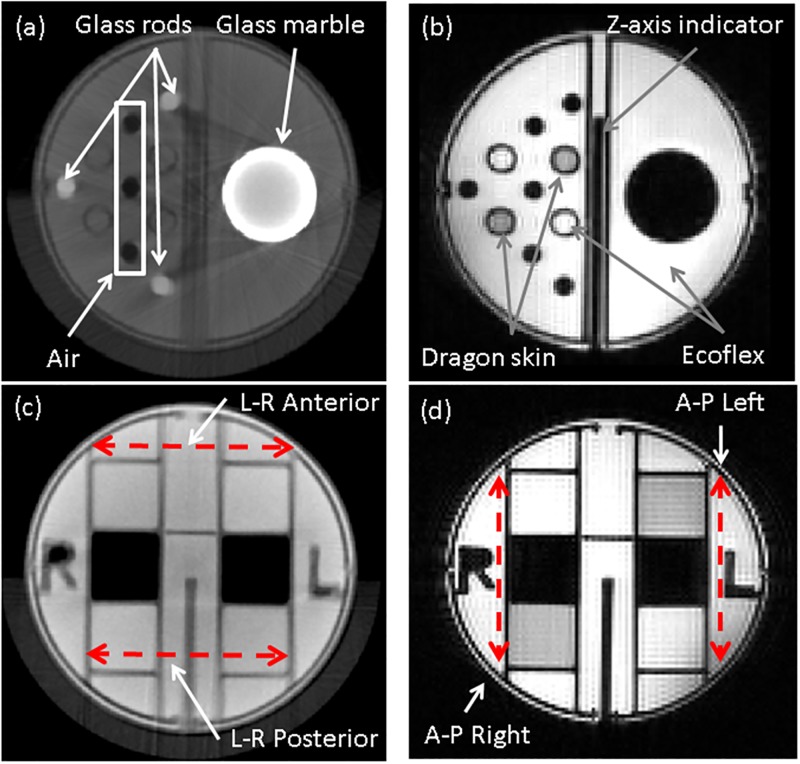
CBCT and the corresponding distortion corrected MR images. (a) CBCT and (b) MR of the contouring and contrast modules. (c) CBCT and (d) MR of the grid module.
